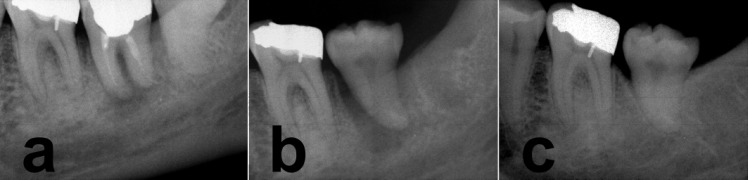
a) Initial periapical radiograph. The second mandibular tooth had vertical root fracture and chronic apical periodontitis. The third mandibular tooth was healthy, b) Radiographic image immediately after transplantation and c) Two years after transplantation, bone and PDL regeneration was observed. Root resorption was not observed.
